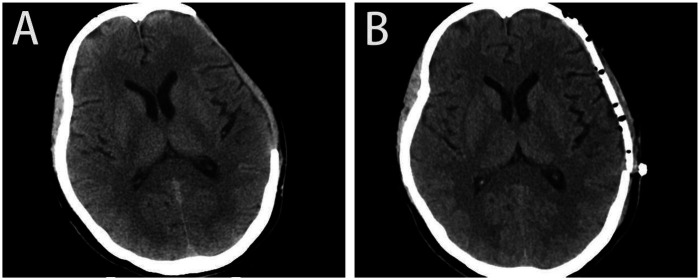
(A): preoperative CT showing left frontotemporal skull defect. (B): Postoperative CT showing well-positioned PEEK implant without intracranial hemorrhage/effusion.
